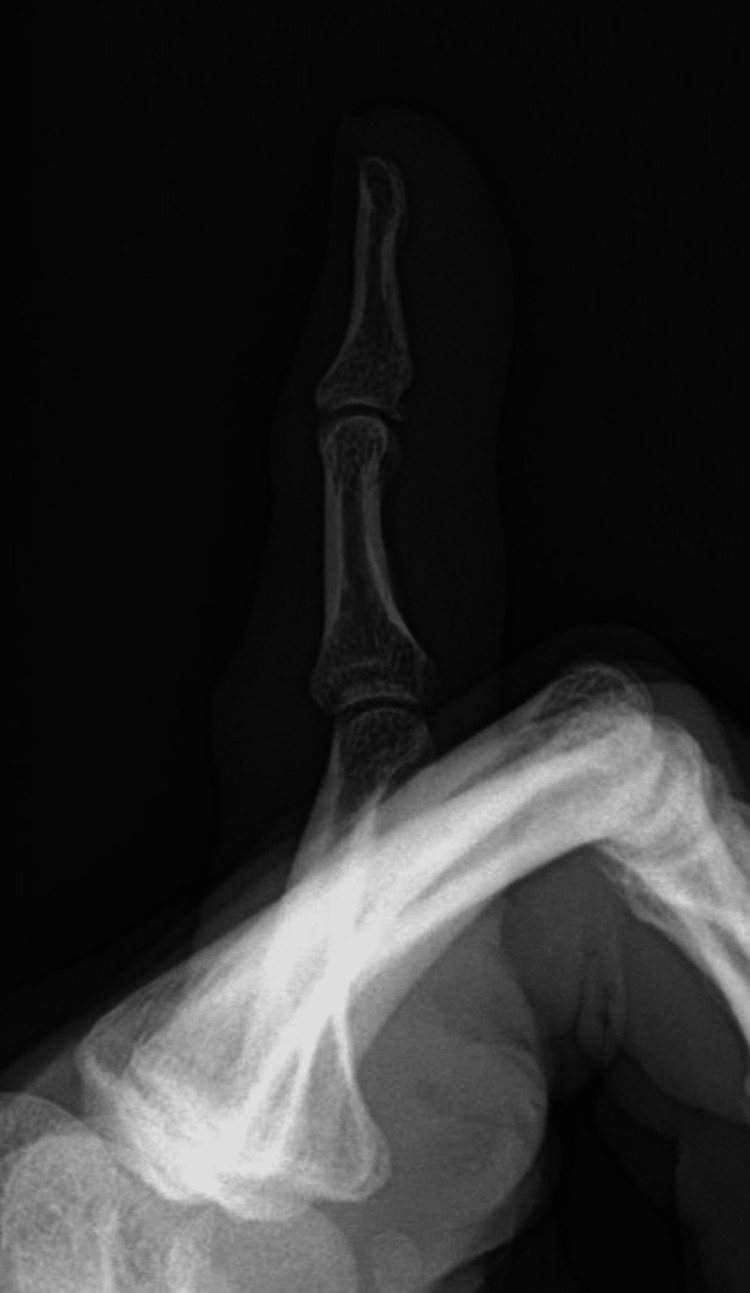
Lateral left little finger radiograph after reduction. Lateral radiograph of the patient’s left little finger after reduction showing the proper alignment of both joints.
